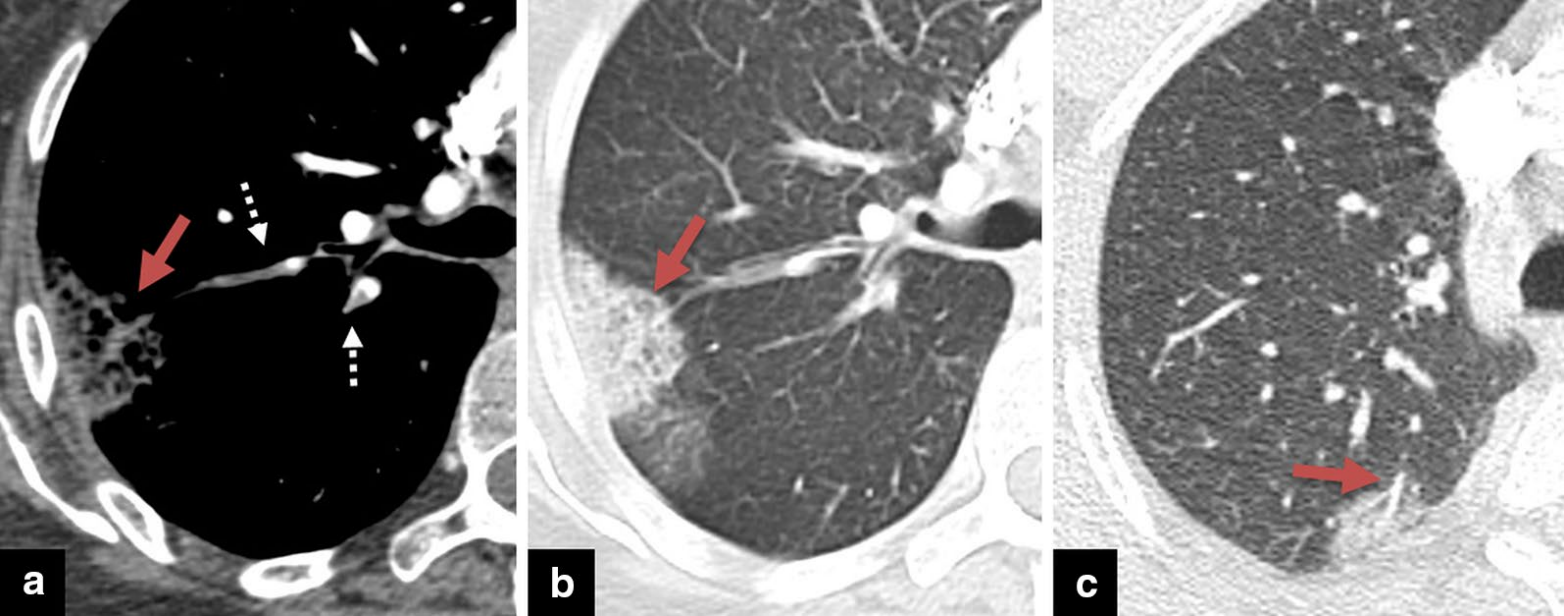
Pulmonary infarction and COVID-19 pneumonia. a Axial CTA image in the mediastinal window shows thrombi in pulmonary artery branches (white arrows). b In the same patient, pulmonary infarction is seen as a subpleural wedge-shaped opacity in the right lung (arrow). c Pulmonary CT angiography was obtained in a patient presented with shortness of breath to the emergency department. CT angiography showed subpleural opacities, but there was no thrombus in the related pulmonary artery branch (arrow). The patient’s COVID-19 RT-PCR result turned out to be positive
